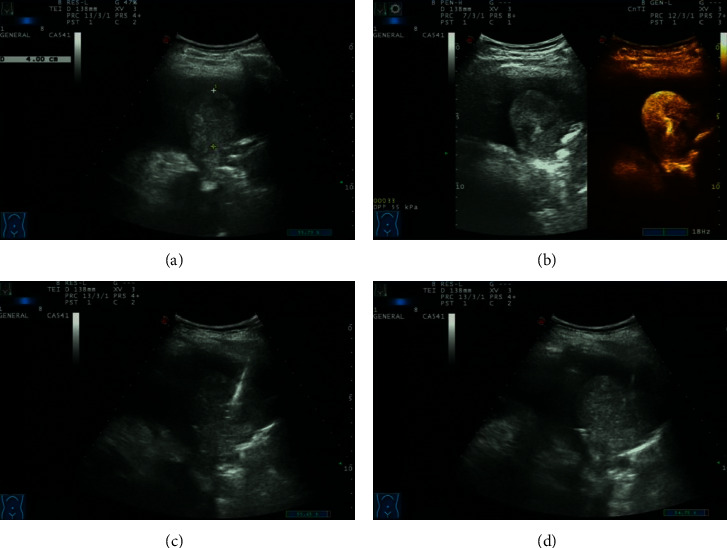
A patient in the contrast-enhanced ultrasound group. (a) Ultrasound examination showed a hypoechoic mass in the lower left lung, 43 mm ∗40 mm in size, with an uneven echo. (b) After injection of the contrast agent, it showed uneven enhancement. (c) The puncture needle was passed through the pleura, into the lesion, and cut for biopsy. (d) Five minutes after needle extraction, no obvious bleeding and pneumothorax and other complications were found.
